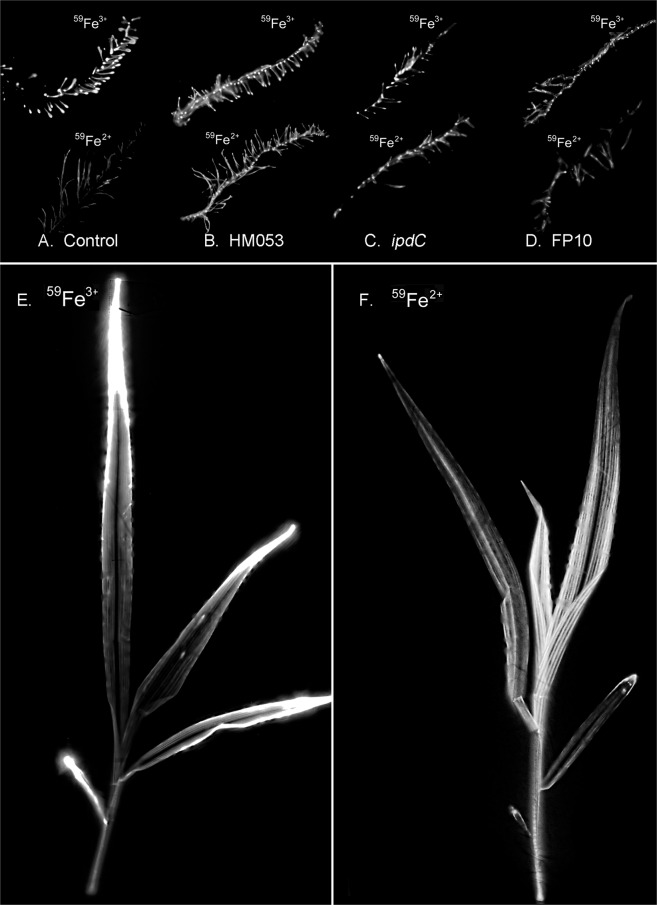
Radiographic imaging of plant tissues reveal different spatial patterning of radioactive ^59^Fe as ferric (Fe^3+^) and ferrous (Fe^2+^) ionic forms. Comparisons were made between non-inoculated control roots (A) and roots inoculated with HM053 (B), *ipdC* (C) and FP10 (D) strains of *A. brasilense* with ^59^Fe^3+^ images are shown in the upper portion of each panel while ^59^Fe^2+^ images are shown in the lower portion. ‘Hot’ spots of ^59^Fe radioactivity (both for ^59^Fe^3+^ and ^59^Fe^2+^) are seen at lateral root junctions. Roots inoculated with the FP10 strain of *A. brasilense* only showed ^59^Fe^3+^ ‘hot’ spots. Shoot images for HM053 inoculated plants are shown for ^59^Fe^3+^ (E) and ^59^Fe^2+^ (F). Translocation of ^59^Fe^3+^ to shoots resulted in higher tracer accumulation in all of the leaf tips. Translocation of ^59^Fe^2+^ to shoots resulted in a higher distribution of tracer in the younger developing leaf tissue.
